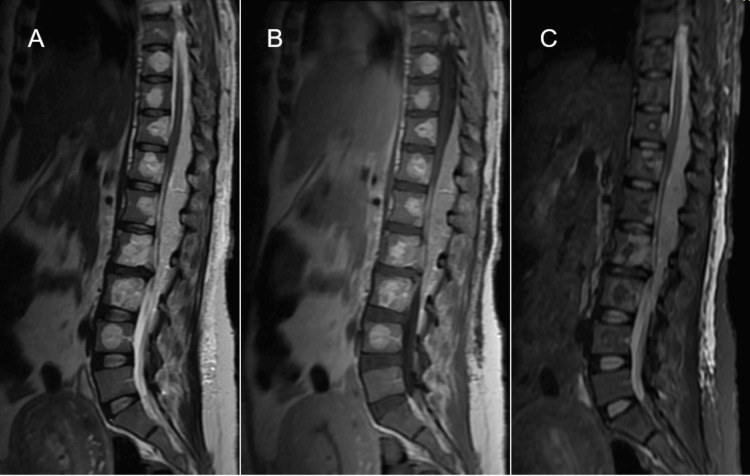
Sagittal thoracolumbar MRI (A) T2-weighted sequence showing hyperintense epidural mass extending from T10 to L3 and invading the vertebral bodies from T9 to L4, causing the effacement of the thecal sac and the compression of the spinal cord. (B) T1-weighted image showing hyperintense lesion in relation to the spinal cord. (C) STIR-weighted MRI showing a hyperintense image with respect to the spinal cord and a hypointense image with respect to the cerebrospinal fluid MRI, magnetic resonance imaging; STIR, short tau inversion recovery
